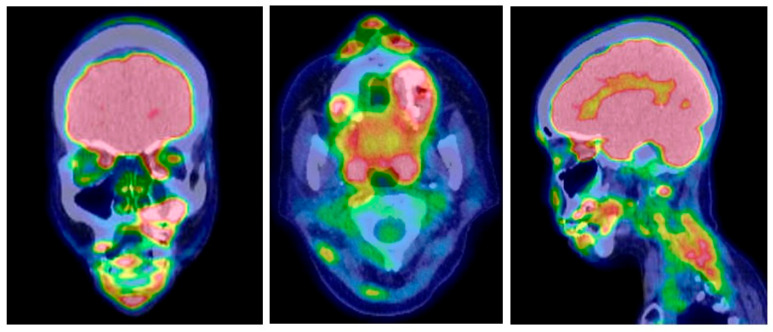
PET/CT findings of the maxillary and mandibular bone lesions at pre-targeted therapy, showing left maxilla and mandible involvement.
